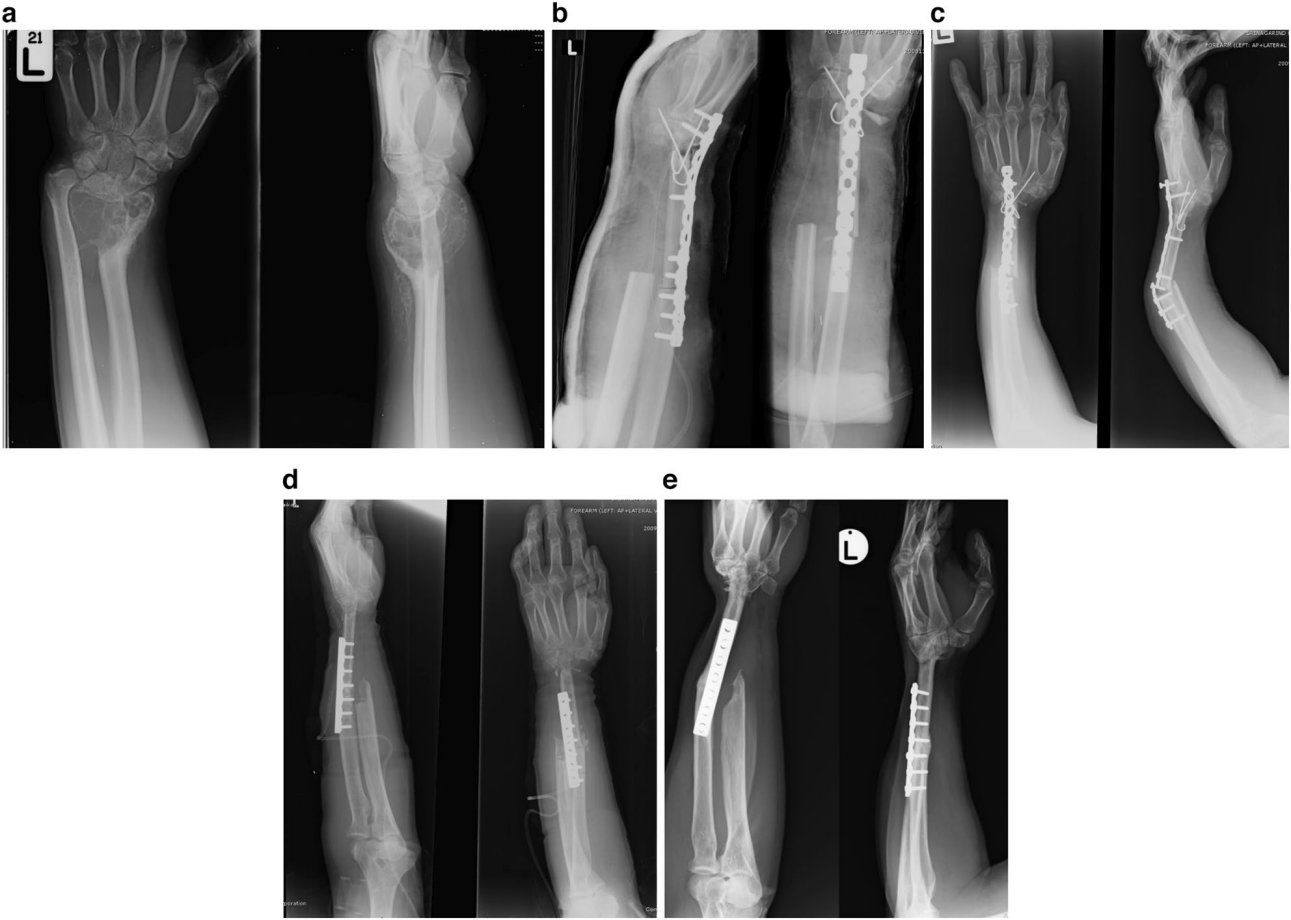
A 47-year-old male with giant cell tumor of the distal radius. (a) preoperative anteroposterior and lateral radiograph. (b) Postoperative radiograph after wide resection and reconstruction with nonvascularized fibula graft and fusion of the wrist joint. (c) Break of plate occurred 13 months postoperatively. (d) Revision surgery with plate and screws plus iliac bone graft, bone union was achieved in 9 months. (e) Three years after the revision surgery with acceptable functional outcome.
